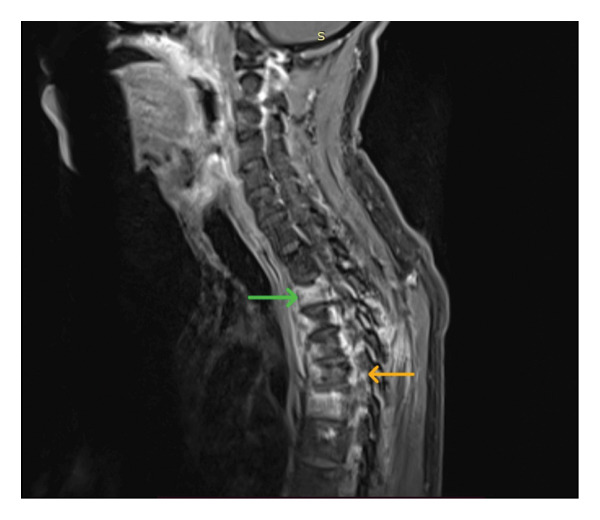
Sagittal T2‐weighted MRI of the spine demonstrating features consistent with infective spondylitis. The green arrow at the T2 vertebral body indicates marrow edema (T2 hyperintensity), while the orange arrow at the T5 level demonstrates spinal canal narrowing with resultant spinal cord compression and associated myelopathic signal change.
